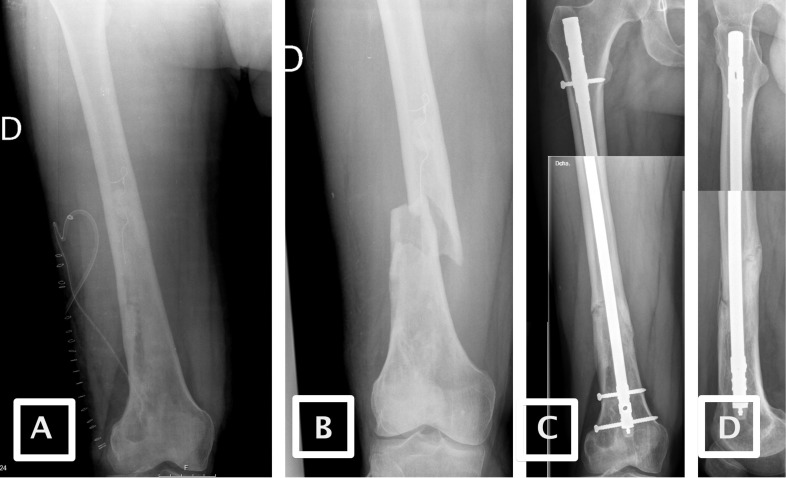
Image A: Postoperative image of the debridement of a sequestrum. Image B: Acute postoperative fracture after debridement. Images C and D: Anteroposterior and lateral X-ray after fixation.
